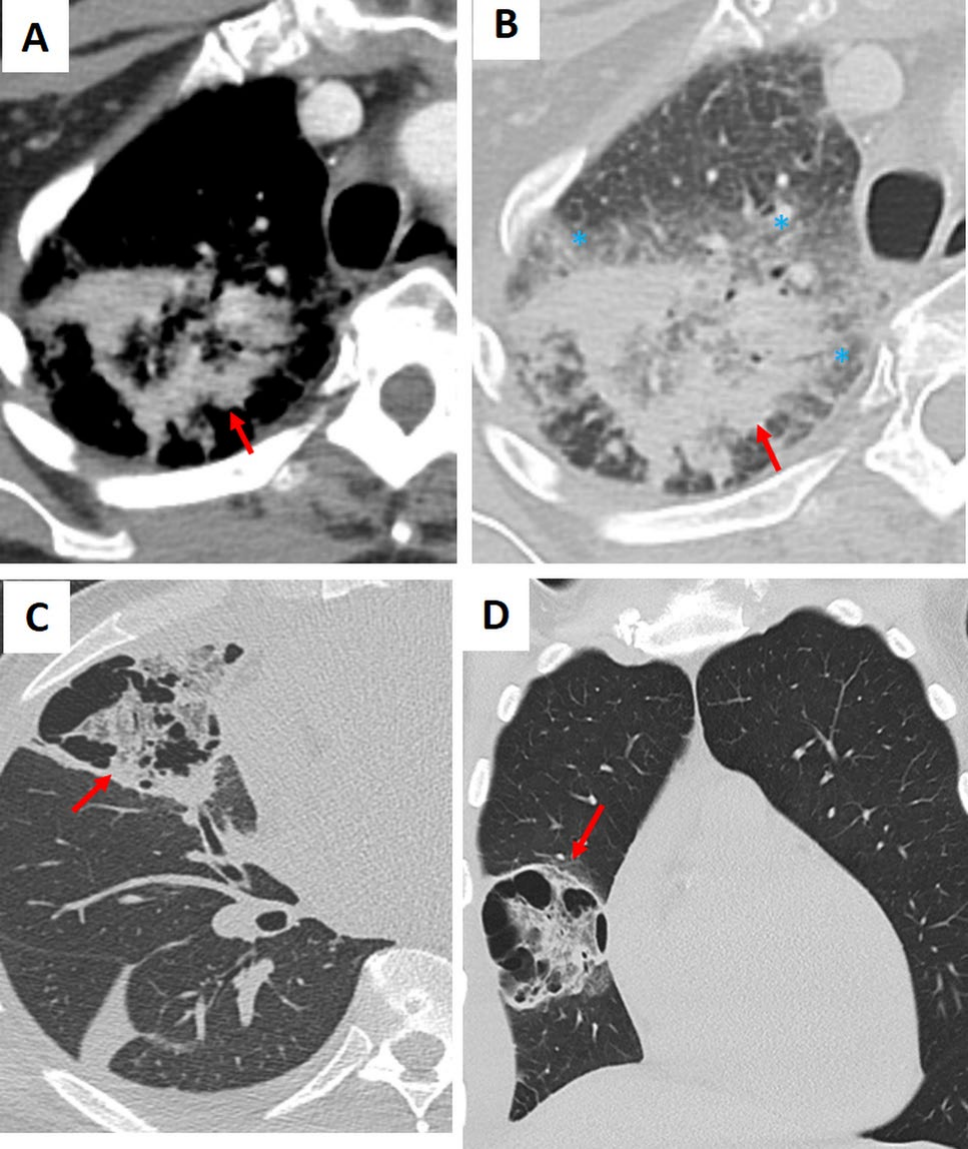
Reverse halo (atoll) sign (arrows) in two patients with primary lung carcinoma. A, B A patient with lung squamous cell carcinoma: Axial (A) mediastinal and (B) lung window CT images show an irregular, spiculated right upper lobe mass with the atoll sign (arrows). Note the surrounding ground-glass opacity (*), which may reflect an invasive component of the tumor. C, D A patient with invasive pulmonary adenocarcinoma: C Axial and (D) coronal chest CT images demonstrate a right middle lobe mass with the reverse halo sign and internal cystic airspaces (arrows)
